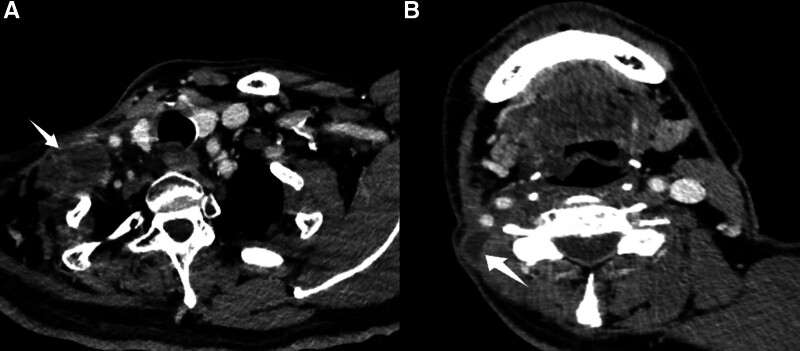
Images of MFS recurrence. (A, B) The enhanced CT showed local recurrence of tumor in the right neck (arrow).
